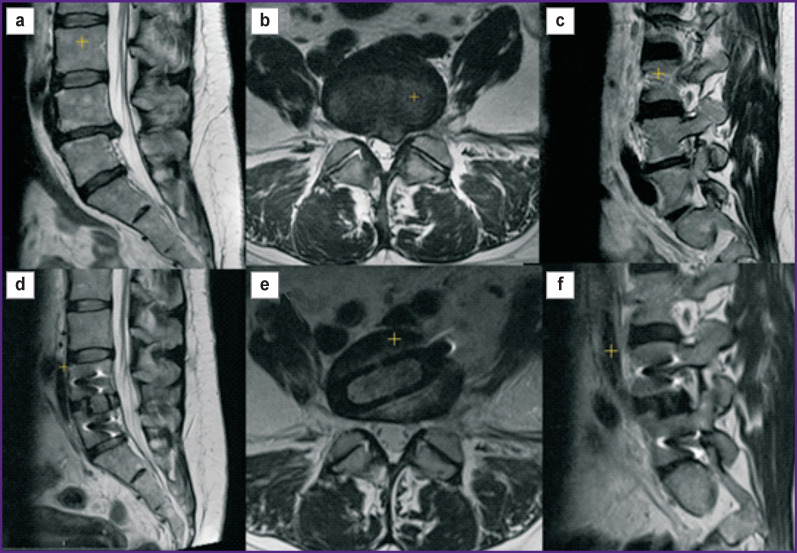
Indirect decompression in OLIF Female patient 52 years old, with recurrent disc herniation at L_4_–L_5_, instability of the L_4_–L_5_ segment, radiculopathy of the L_5_ vertebra on the left, and chronic vertebral pain syndrome. MRI image shows the sagittal section in the central part of the spinal canal: (a) before surgery, (d) after OLIF; axial section through the L_4_–L_5_ disc: (b) before surgery, (e) after OLIF; sagittal section at the level of the left intervertebral foramen: (c) before surgery, (f) after OLIF
